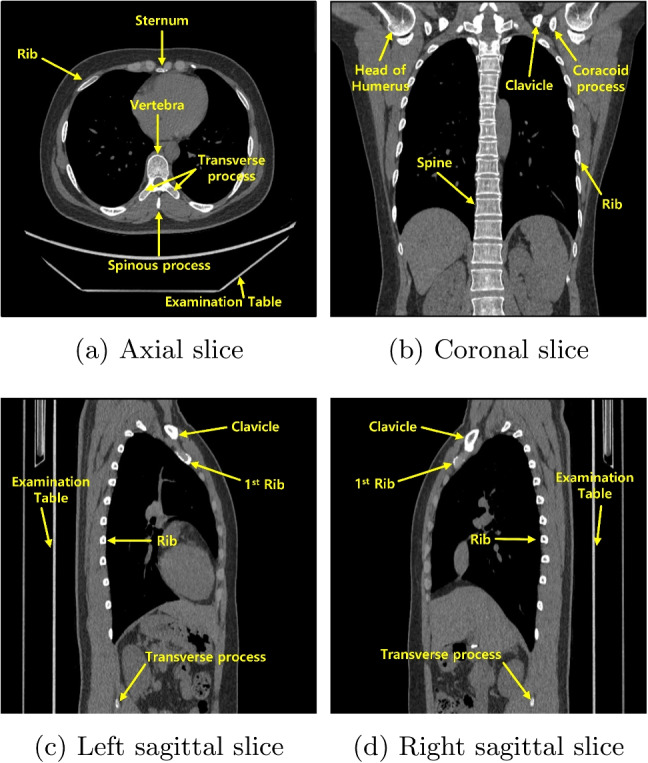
Representative examples of multiplanar slices of chest CT. While axial and coronal views contain overlapping structures that hinder rib isolation, the sagittal slices clearly delineate all twelve rib pairs with minimal anatomical interference, offering an optimal perspective for rib localization and segmentation
